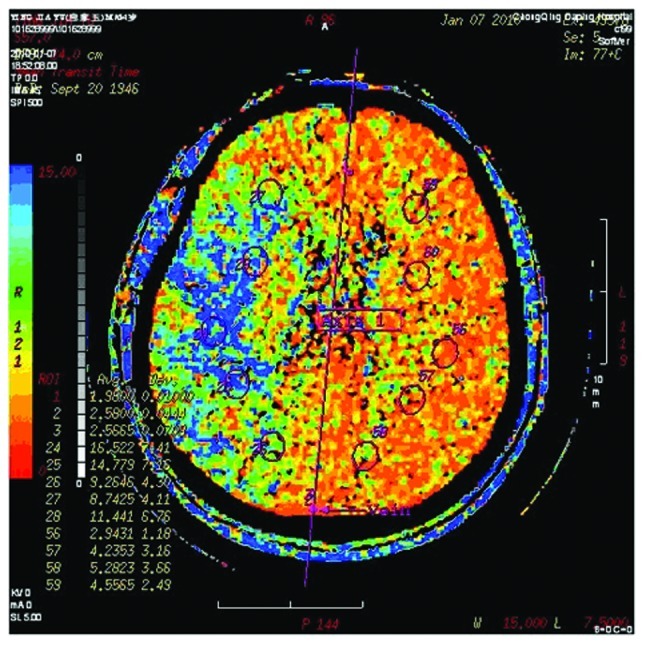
Brain perfusion computed tomography prior to intervention.
